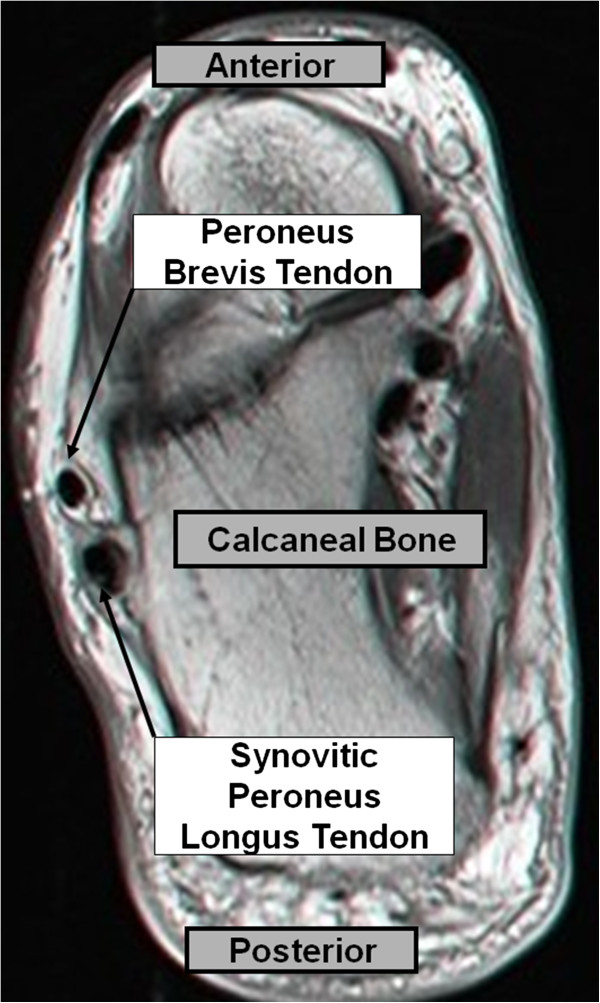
Axial MRI view showing the synovitic thickened peroneus longus.
